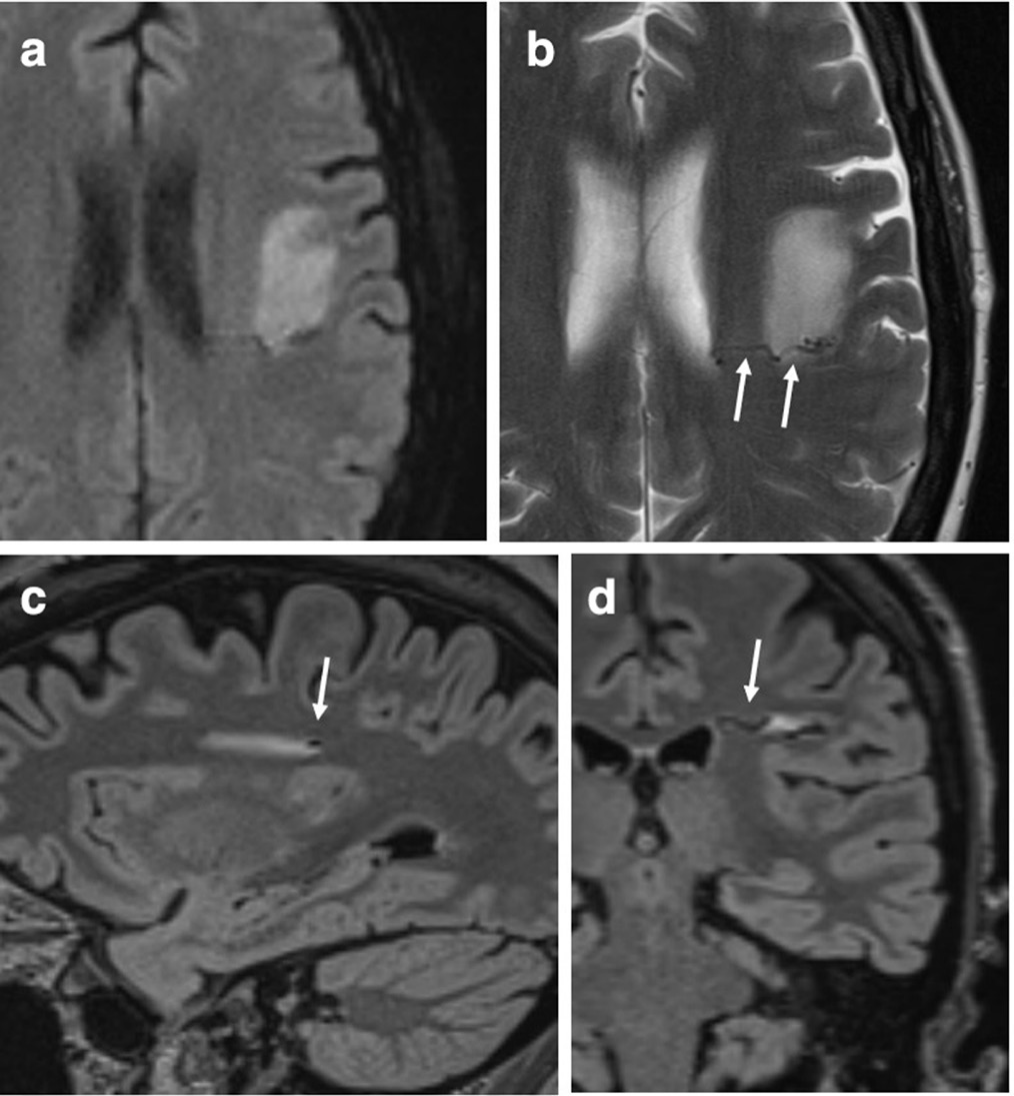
Brain MRI reveals a subacute cerebral infarct in the left frontal white matter as evidenced by increased signal intensity on the diffusion-weighted sequence (A) and decreased ADC values (not shown). On the corresponding axial TSE *T*_2_-weighted image (B) on the sagittal (C) and coronal (D) 3D FLAIR images, along the poster margin of the left frontal white matter infarct a serpiginous draining vein is seen, as identified by a flow void (white arrows).
